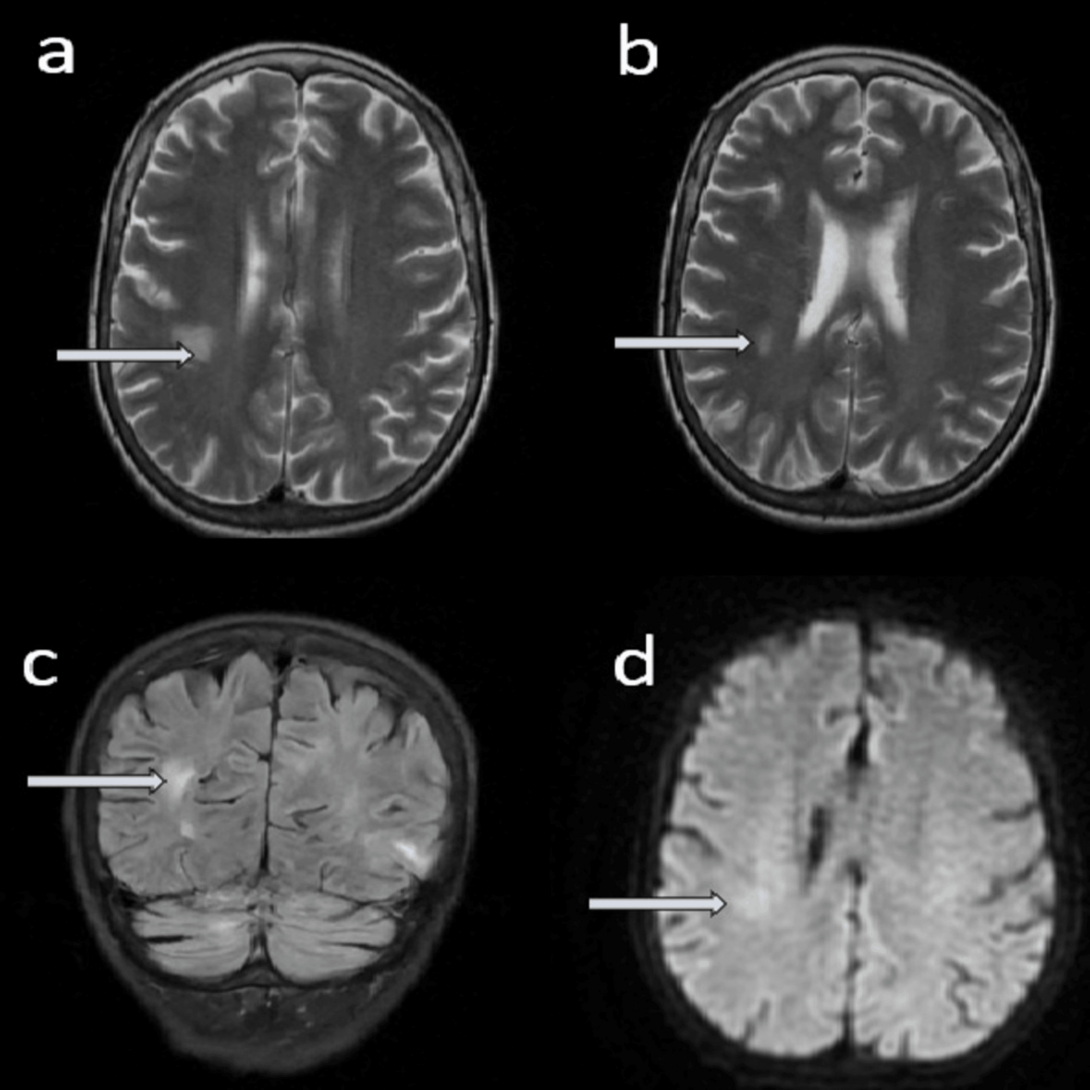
MRI head without contrast revealing radiological features of posterior reversible encephalopathy syndrome (PRES). (a) and (b) T2W images-axial section, (c) FLAIR view-coronal section, and (d) DWI image-axial section. DWI: diffusion-weighted imaging; FLAIR: fluid-attenuated inversion recovery.
